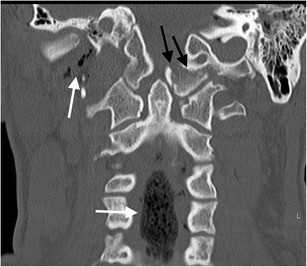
Type II occipital condyle fracture: coronal reformatted CT image of the craniocervical junction performed as part of a CT traumogram in a young male pedestrian struck by a bus. The fracture of the left occipital condyle is associated with extension into the right basiocciput (*black arrows*). Note the associated soft tissue emphysema and pneumorachis within the anterior epidural space of the cervical spine *(white arrows*)
